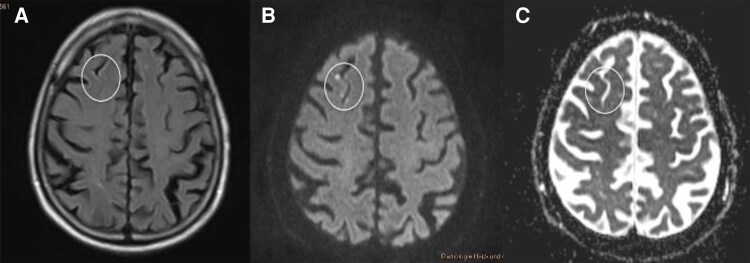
Silent cerebral events documented in a Patient 1 day after AF ablation on cerebral MR imaging: *A*) FLAIR sequence (no lesions), *B*) DWI with hyperintense lesions, and *C*) ADC map with corresponding hypointensity [differentiation between SCEs (FLAIR negative) and SCLs (FLAIR positive)]. ADC, apparent diffusion coefficient; DWI, diffusion-weighted imaging; FLAIR, fluid-attenuated inversion recovery; MR, magnetic resonance; SCL, silent cerebral lesion; SCE, silent cerebral events.
